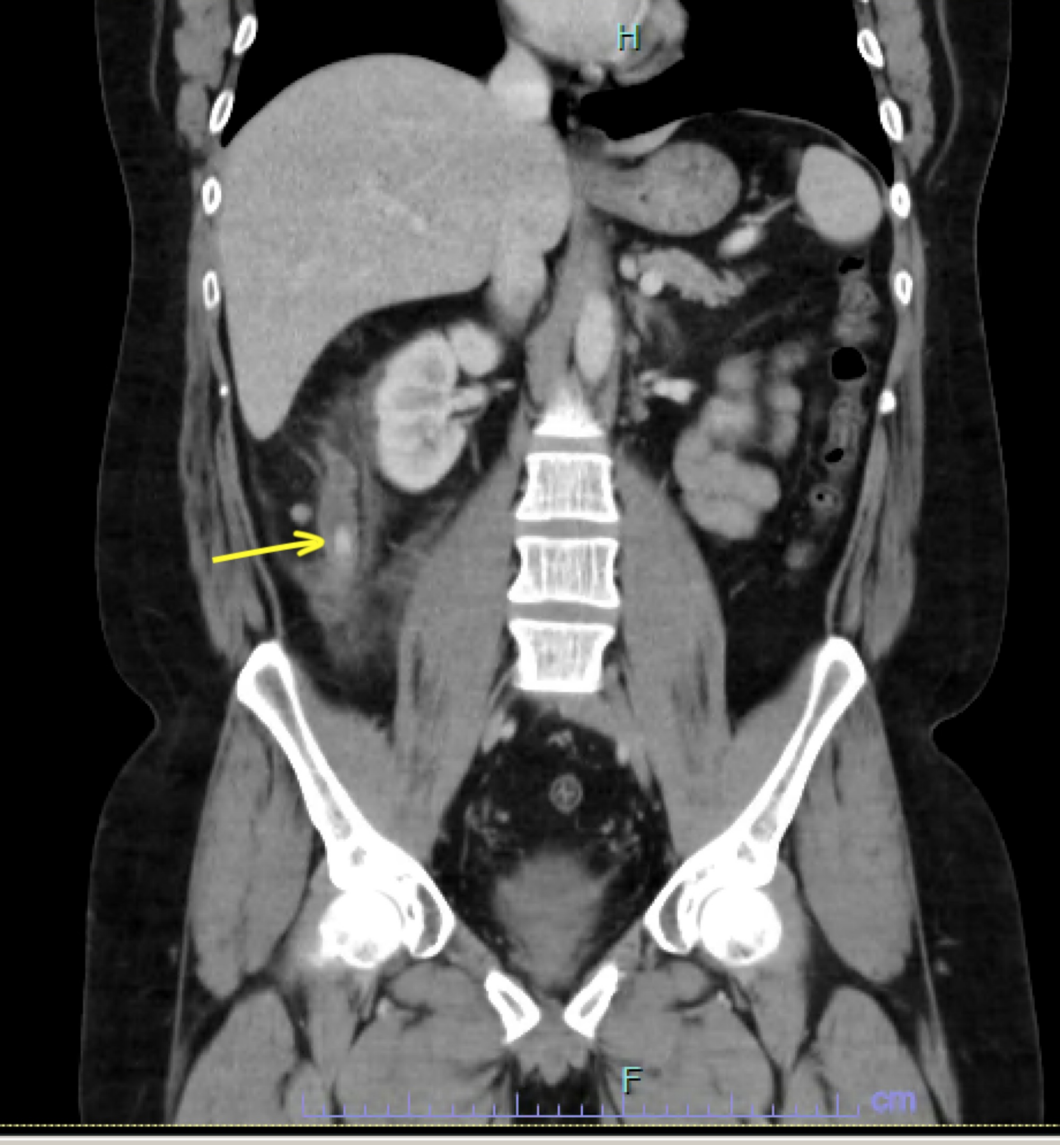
CT scan of the abdomen and pelvis showing acute appendicitis and an 11-mm appendicolith at the base of the appendix
